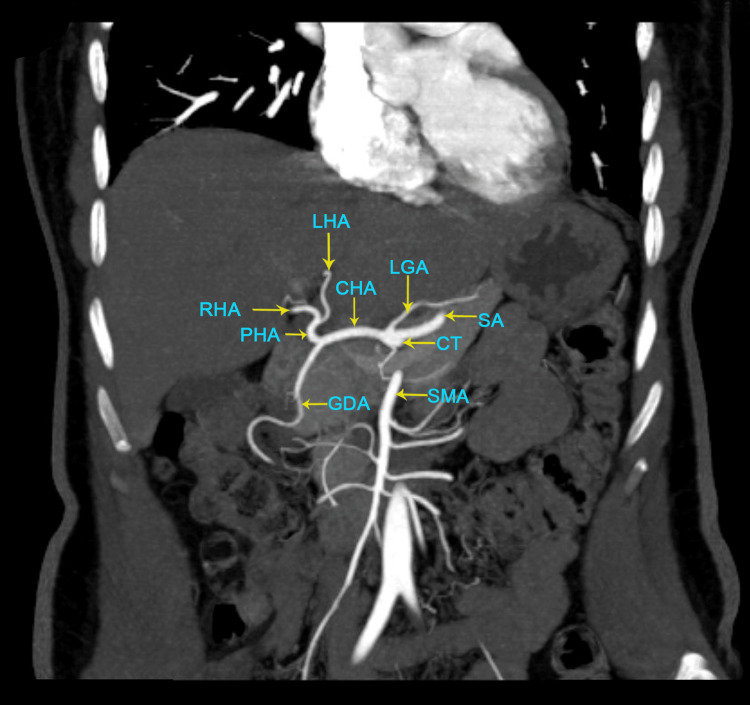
MDCT coronal MIP image of the normal hepatic arterial anatomy MDCT: multidetector computed tomography; MIP: maximum intensity projection; LHA: left hepatic artery; RHA: right hepatic artery; CHA: common hepatic artery; PHA: proper hepatic artery; LGA: left gastric artery; SA: splenic arteries; CT: celiac trunk; GDA: gastroduodenal artery; SMA: superior mesenteric artery (The figures are from the patients included in the current study.)
